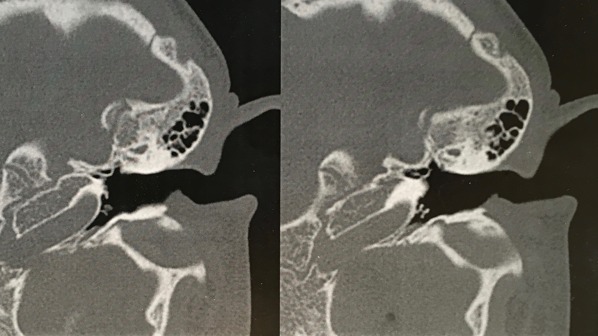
CT showing the presence of a tympanostomy tube in the left middle ear space
